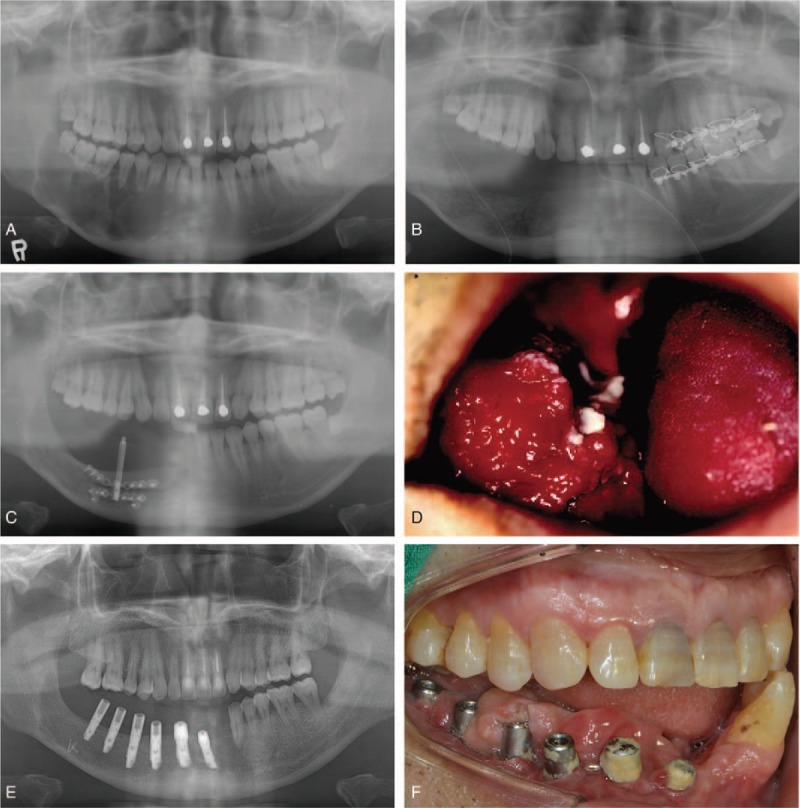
Surgical phase. Panoramic radiographs (A) of the ameloblastoma at the right side of the mandible, (B) of the ameloblastoma after mandibulotomy and nerve repositioning, and (C) of the reconstructed mandible with the distractor. (D) Recurrence of the ameloblastoma. (E) Panoramic radiograph of the mandible after implant placement. (F) Lateral view of the mandible. There was no available space due to the overgrown mucosa. Poor oral hygiene was observed at the healing abutments.
